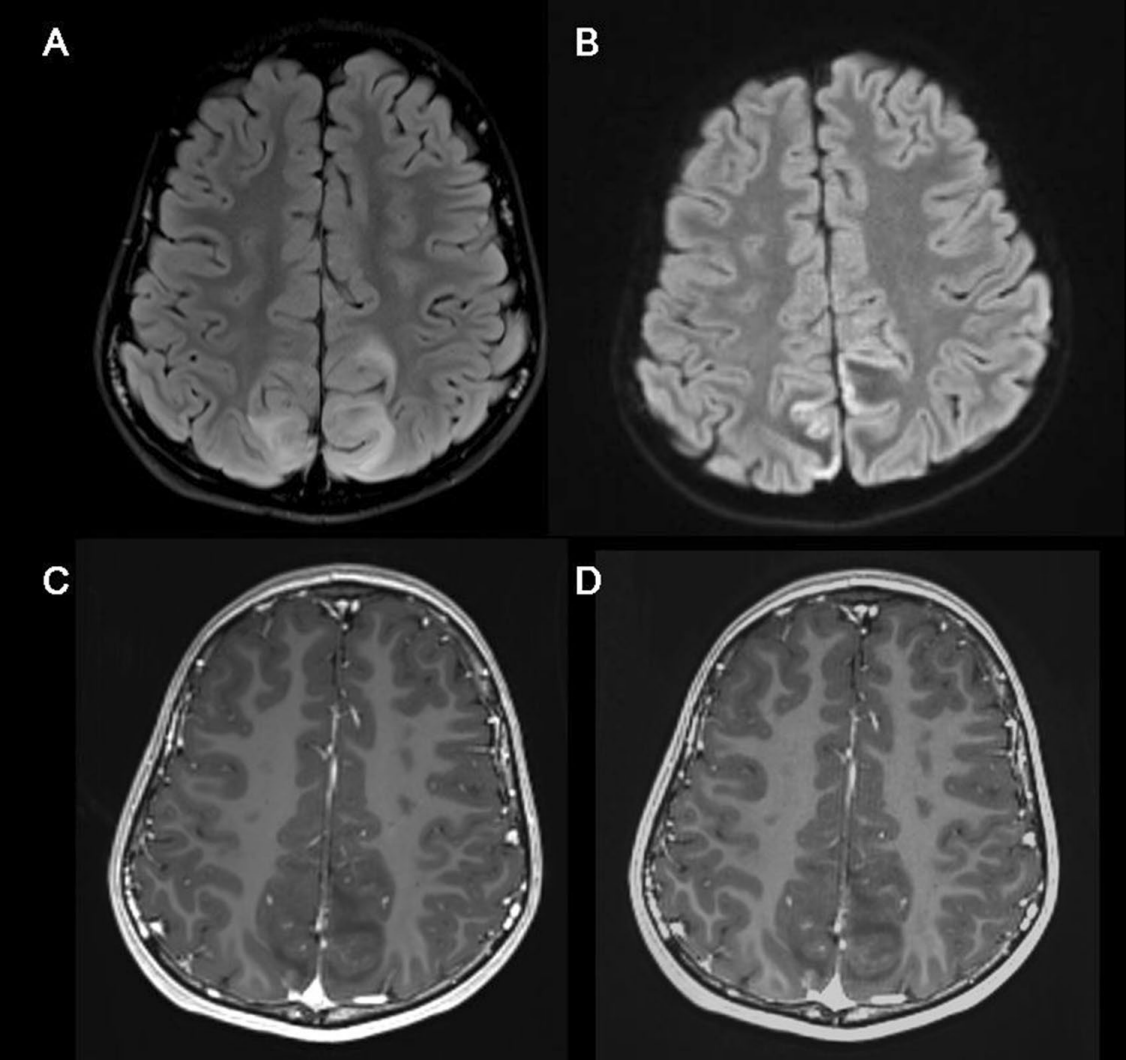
Probable encephalitis on conventional and wave-CAIPI MPRAGE. A boy aged 8 years and 2 months with convulsion-like movements and motor weakness of the lower limb, and fever shows confluent FLAIR high signal lesions in both parieto-occipital lobes (A) with some diffusion restriction in the cortexes (B). After contrast injection, there is no evidence of enhancement on both conventional MPRAGE (C) and wave-CAIPI MPRAGE (D). Based on the images and clinical and laboratory findings, the patient is diagnosed with encephalitis.
